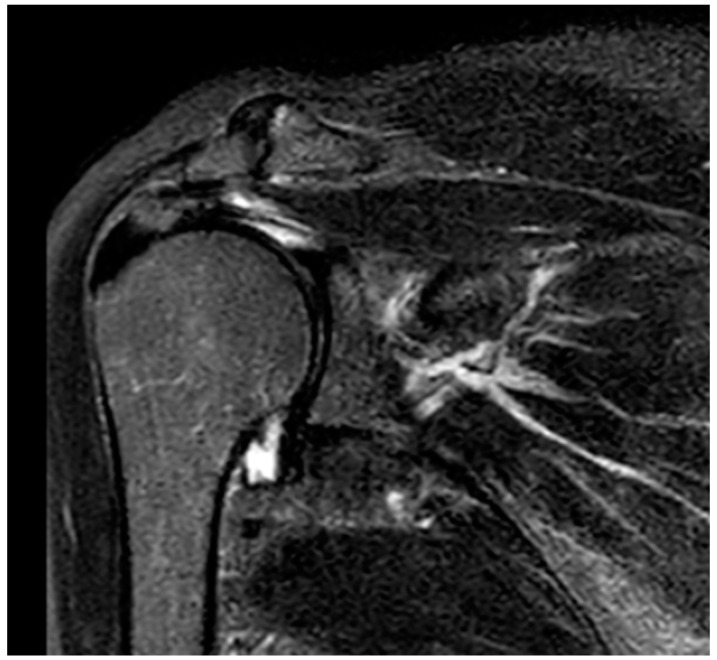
The pre-discharge MRI shows an osteofibrous sleeve of the acromion-clavicular joint with impingement of the supraspinatus tendon with a full-thickness anterosuperior lesion.
